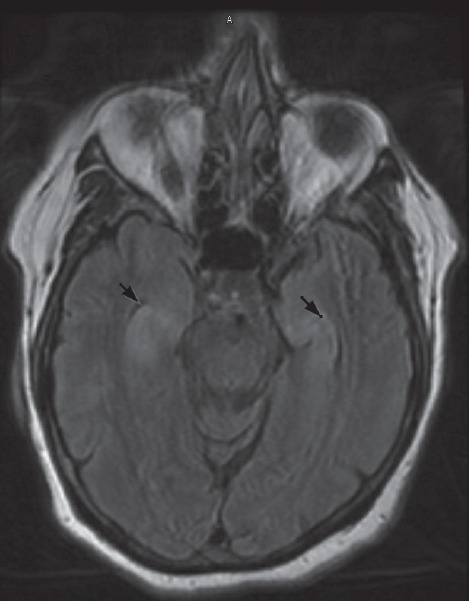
Brain MRI revealing symmetric signal abnormality, swelling of the hippocampus and right mesial temporal lobe, and a T2 FLAIR signal abnormality.
